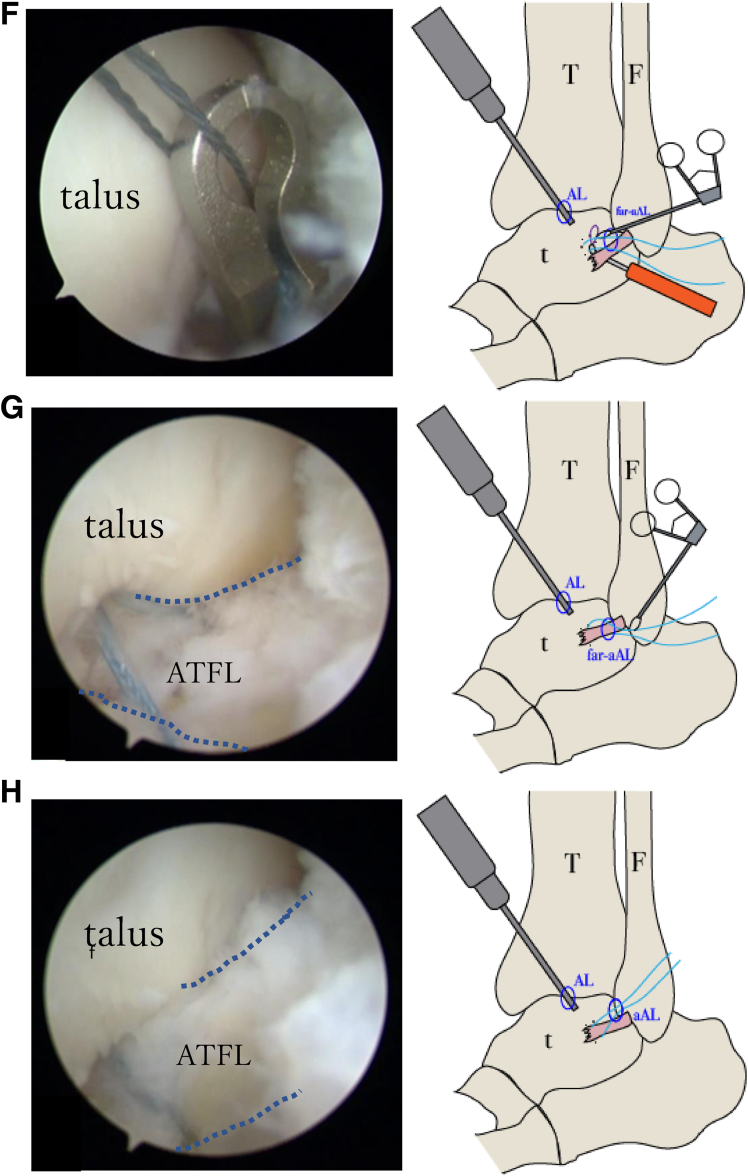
Intraoperative findings. The accessory anterolateral (aAL) portal is created as a working portal, and the anterior talofibular ligament (ATFL) is evaluated using the anterolateral (AL) portal as the viewing portal. (A) Assessment of ligament quality using a probe reveals no damage or degeneration of the ATFL in the fibular region adjacent to the ligament attachment. (B) When viewed from the AL portal, the ATFL is torn on the talar side, accompanied by small bone fragments (yellow arrow). Bone fragments are removed from the aAL portal. (C) On the basis of the markings created using ultrasonography, the footprint (yellow oval) on the talar side is confirmed under imaging using an 18-gauge needle using the AL portal as the viewing portal, and a far a AL portal is placed directly above the footprint of the talar ligament attachment.. (D) We drill into the talar footprint in the direction of the medial malleolus (red arrows) using the AL portal as the viewing portal and insert a suture anchor at the talar insertion of the ATFL through the far aAL portal. (E) The ATFL (blue dotted lines) is lifted with a needle, and a guidewire is inserted from the far aAL portal across the ATFL using the AL portal as the viewing portal. (F) The anchor thread is passed through the guidewire loop, and sutures are passed through the ligament from the far aAL portal. (G, H) The threads are concentrated in the aAL portal and sutured in the dorsiflexion position. The ATFL (blue dotted lines) is fixed without loosening. (F, fibula; t, talus; T, tibia.)
